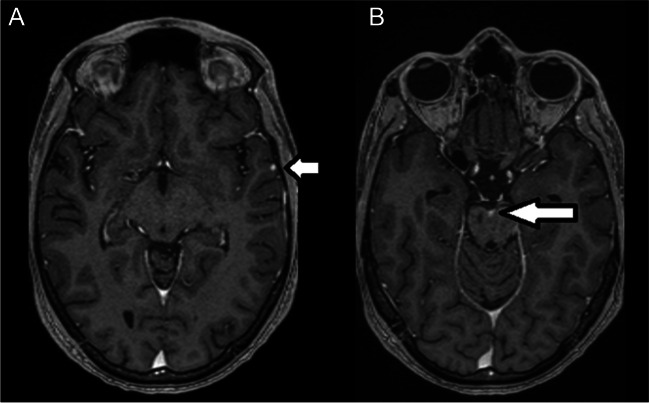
Gadolinium-enhanced MRI T1 (MP-RAGE) showed a focal-enhancing lesion cortical in the left temporal lobe (A) and interpeduncular cistern (B)
